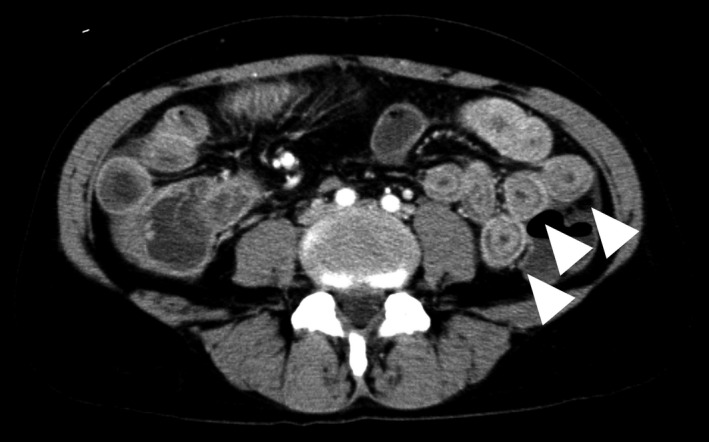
Abdominal contrast‐enhanced computed tomography demonstrating the edematous and thickened small intestinal wall with inner and outer circumferential enhancement known as the target sign (white arrowheads)
